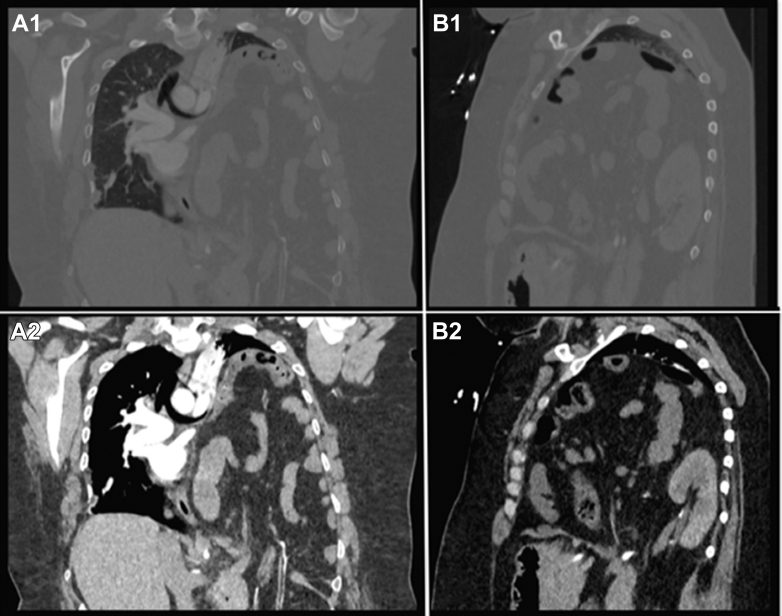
The images are obtained during a computed tomography pulmonary embolism study. Images marked with an “A” and “B” show the coronal and sagittal view of the patient’s chest, respectively. Images marked with a “1” and “2” are in the lung and abdominal view, respectively. The images show a chronic Bochdalek hernia. Near-complete atelectasis of the left lung is best seen in images A1 and B1. Image A2 demonstrates herniation of the visceral fat, bowels, and vascular structures across the diaphragm. Image B2 demonstrates the posterior diaphragmatic defect resulting in herniation of the bowel and the left kidney.
